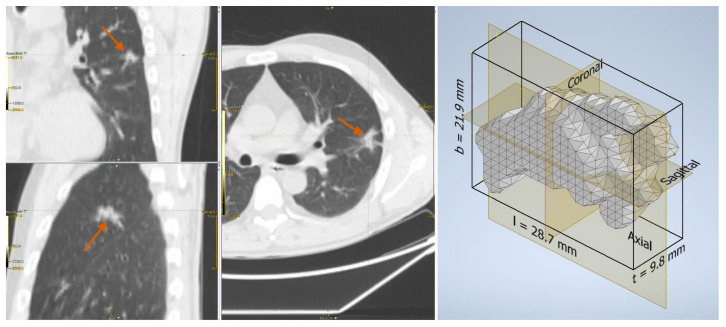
Fibrosis in a 46-year-old man: lesion on the CT scan (left) and the reconstructed three-dimensional volume within the axis-aligned bounding box (right).
